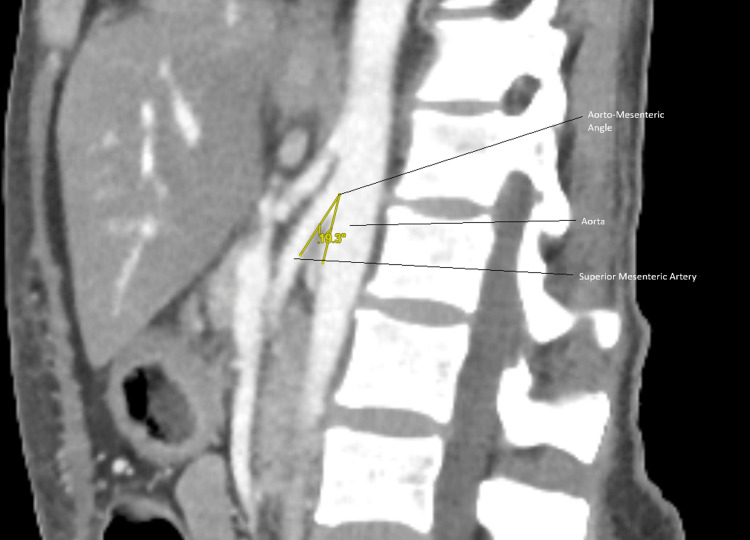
Sagittal contrast-enhanced CT showing a reduced aortomesenteric angle of 19.3°, below the diagnostic threshold of <22°, resulting in compression of the third part of the duodenum and confirming superior mesenteric artery syndrome.
